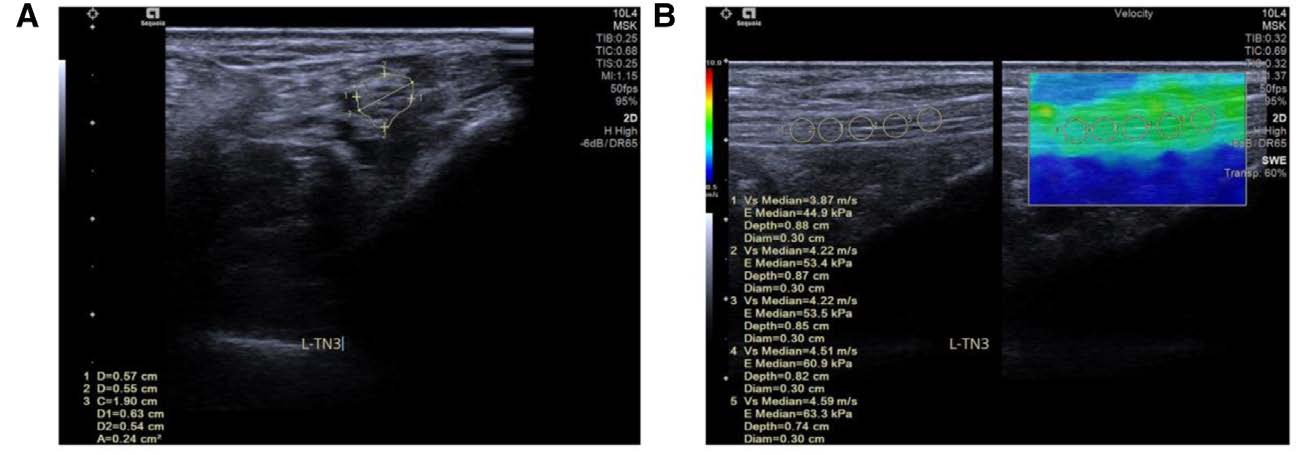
Ultrasound diagnosis. (A) The cross section of the NUS reveals showed tibial nerve CSA was measured to be 0.24 cm^2^; (B) the longitudinal section of NUS showed the perineurium of tibial nerve of the inner ankle is slightly blurred, ROI: 3 mm × 3 mm; measurements taken at medial malleolus. TN: tibial nerve. CSA = cross-sectional area, NUS = nerve ultrasound, ROI = regions of interest.
